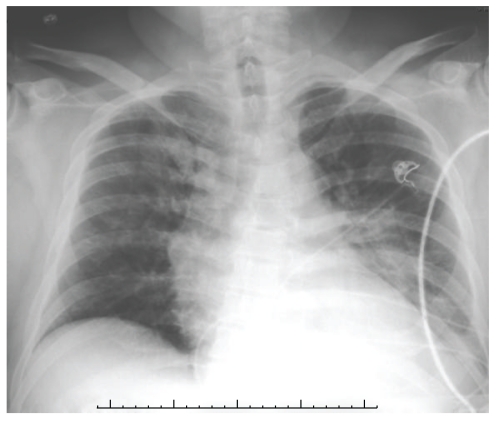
Chest X-ray on day 4 showing resolution of the earlier changes.
